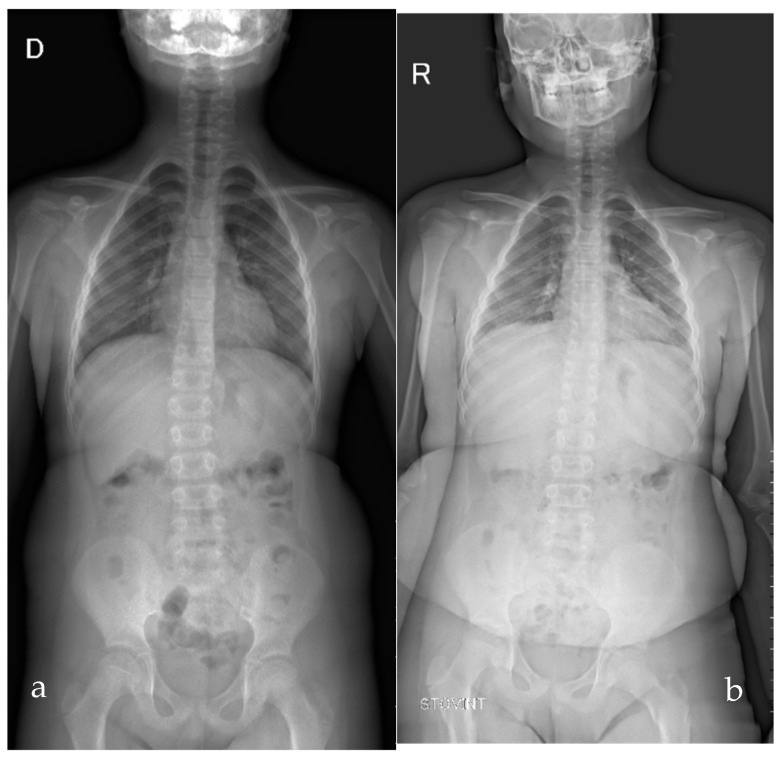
Full-length spinal radiograph, posteroanterior view. (a) 3.5 years post-injury, radiographic assessment revealed a minor thoracolumbar dextroscoliosis measuring ~9° according to the Cobb method. (b) 5 years post-injury, no significant progression of the scoliosis was observed.
